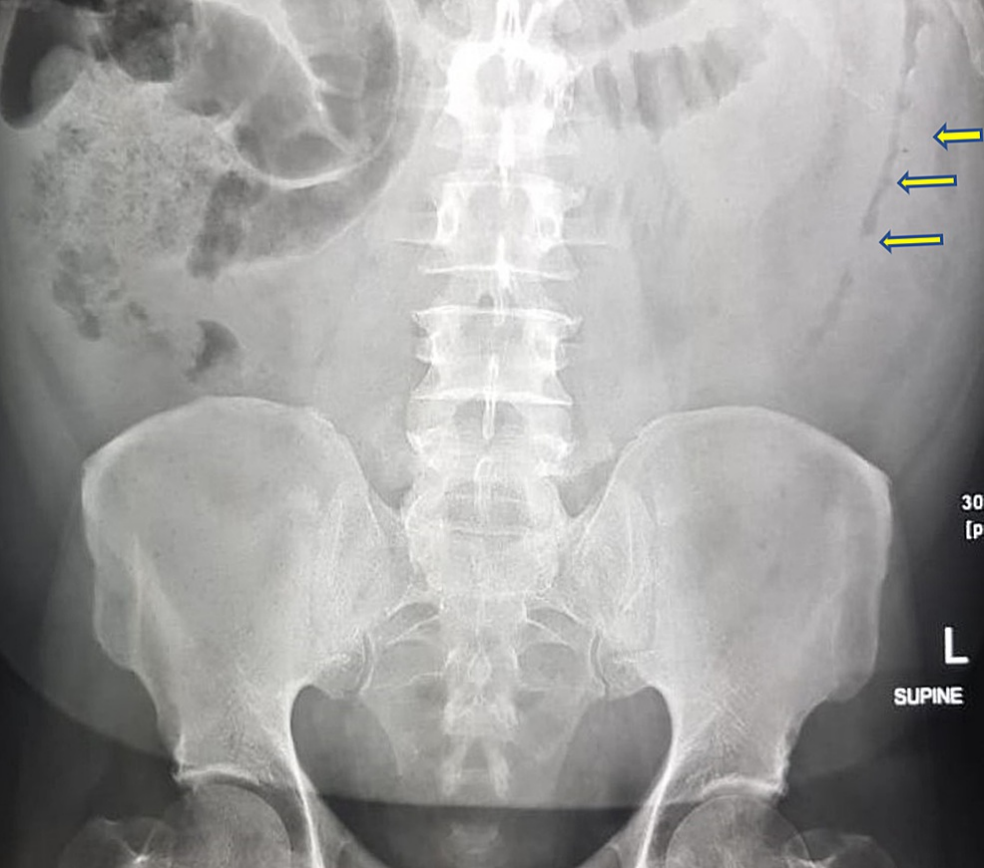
Abdominal x-ray showing mucosal wall thickening with narrowing in the lumen of the descending colon (yellow arrows).
